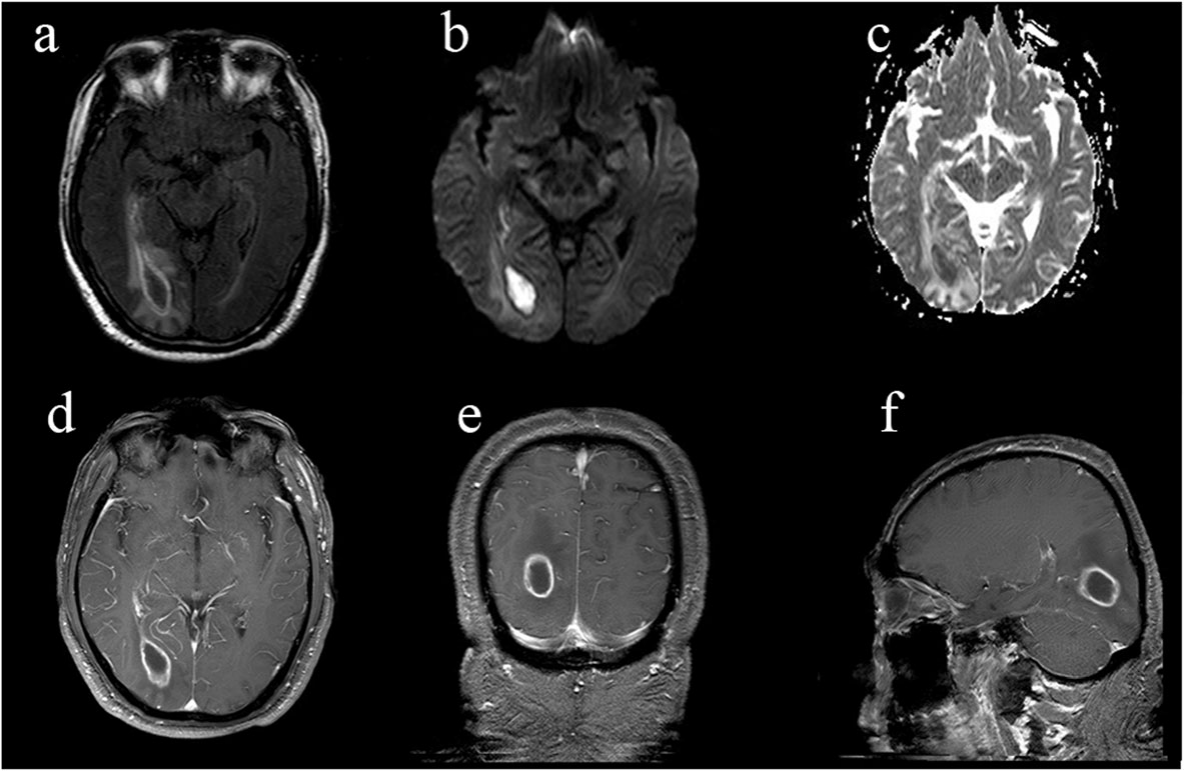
Brain MRI findings. a Axial FLAIR: a mass lesion with perilesional edema adjacent to right lateral ventricle occipital horn; the center of the lesion is hyperintense on DWI (b) and hypointense on ADC maps (c); after gadolinium injection, the lesion has a well-defined, thin-walled enhanced rim(d-f), which is consistent with the image findings of brain abscess. DWI, diffusion-weighted image; ADC, apparent diffusion coefficient
